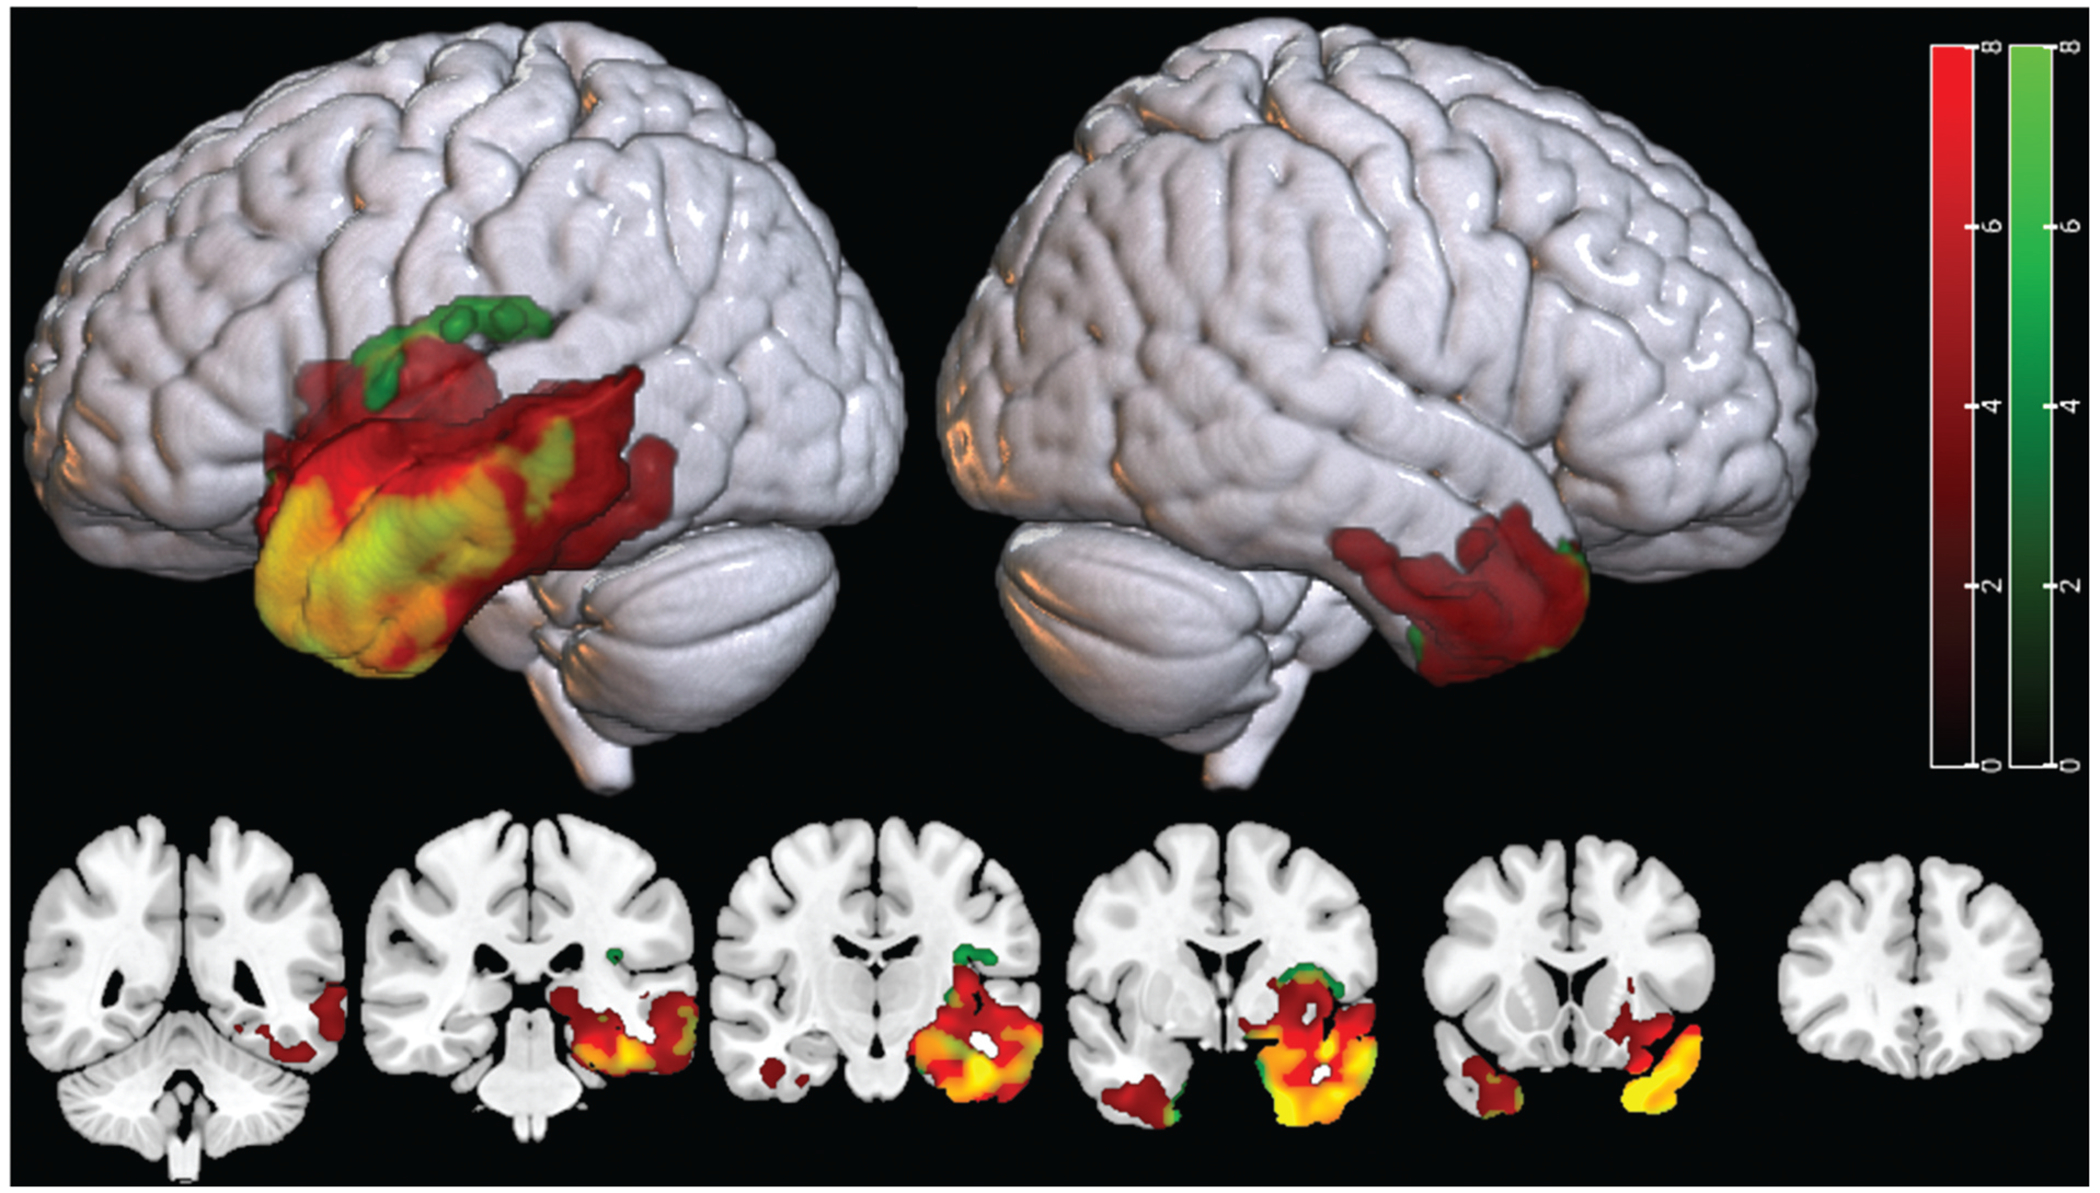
Atrophy (red) and hypometabolism (green) thresholded t-maps for people with semantic PPA (*n* = 11). colored regions indicate significantly (*p* < 0.001, FWEc *p* < 0.05) decreased gray matter (red) or SUVR signal (green) as compared to healthy participants (*n* = 10). Yellow indicates areas with both significant hypometabolism and atrophy.
